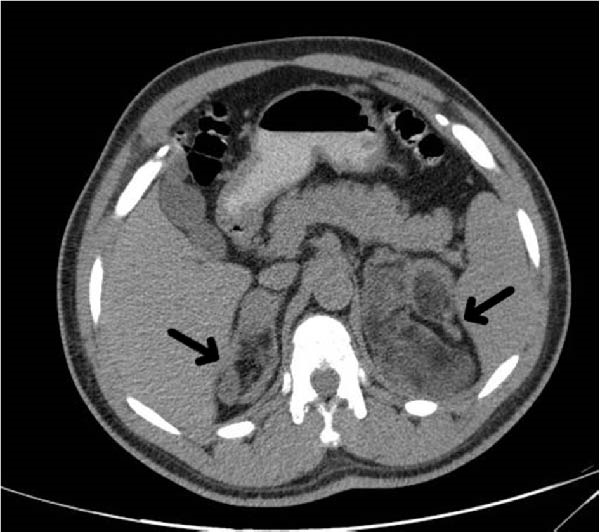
Abdominal CT scan revealing bilateral adrenal myelolipomas: large lesion in the left adrenal (98 mm × 63 mm) and smaller lesion in the right adrenal (49 mm × 24 mm).
